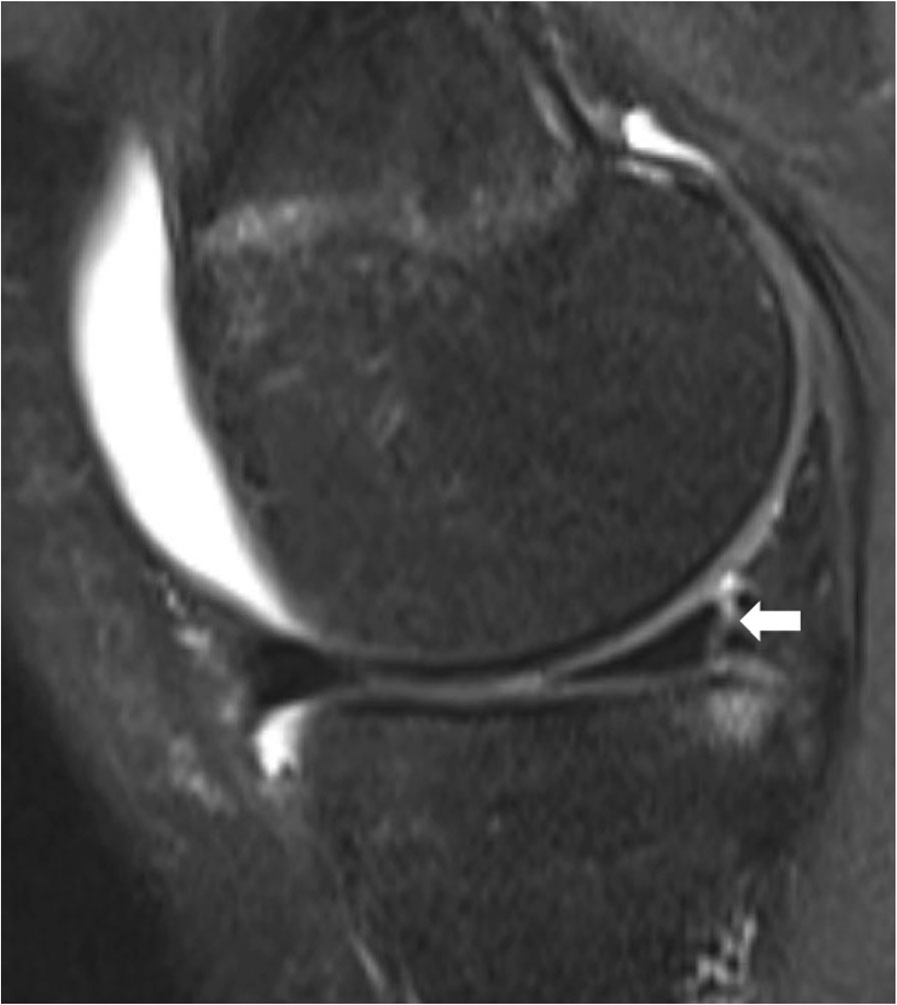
A ramp lesion on a sagittal magnetic resonance imaging exam (MRI). The white arrow points to a peripheral tear in the posterior horn of the medial meniscus, aka a ramp lesion. These lesions are often missed on MRI, and even during arthroscopic examination
